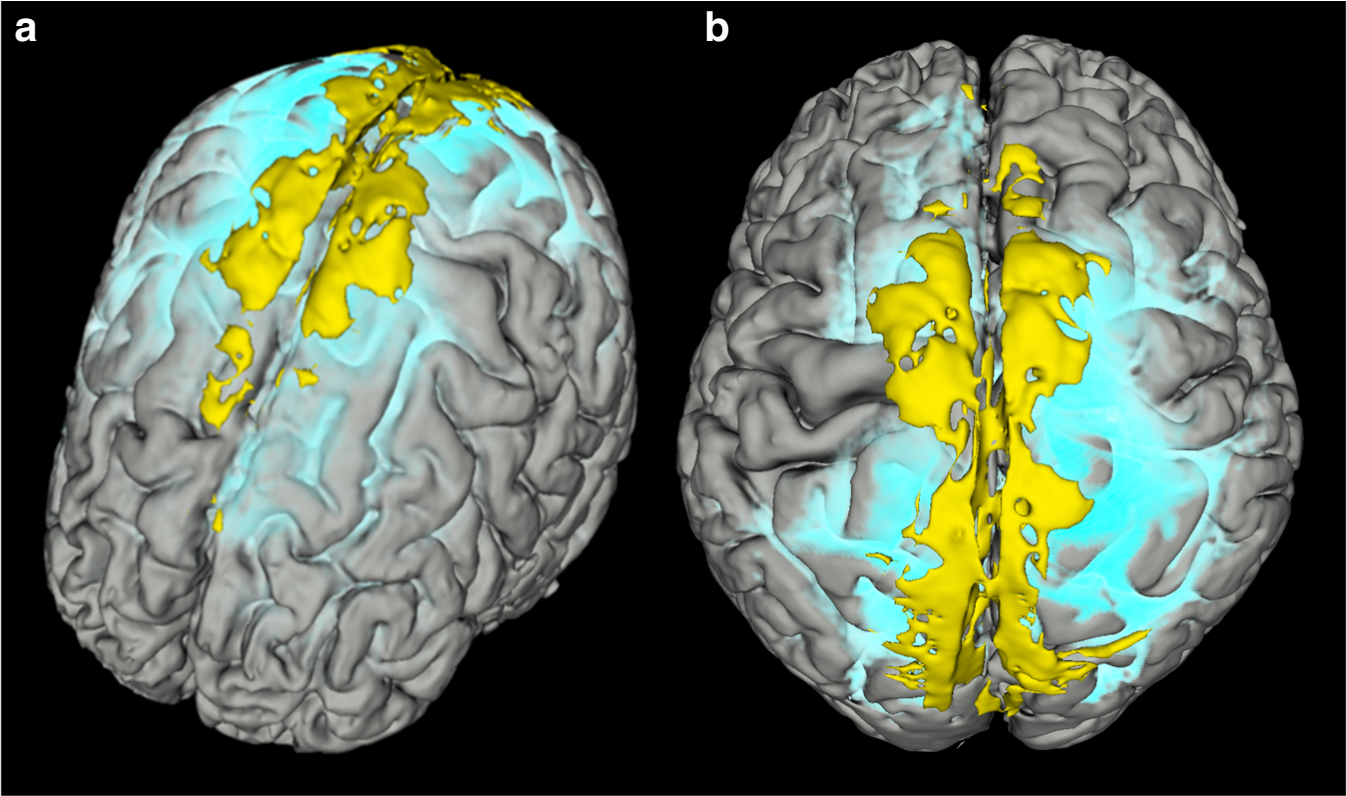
3D representation of parasagittal dura as defined from T2-FLAIR. The brain is shown in oblique coronal view (a) and viewed from above (b). Parasagittal dura (dark yellow) as defined from T2-FLAIR and co-registered with rough segmentation of the brain (gray) and tracer enhancement in CSF (light blue) from T1 GRE at 48 h after intrathecal administration. The CSF tracer propagated toward the upper brain convexities and parasagittal dura at late time points (at 24 h and particularly at 48 h). A video-animation presenting the full longitudinal extension of parasagittal dura is presented in Supplementary Movie 2. Images: Tomas Sakinis, MD.
